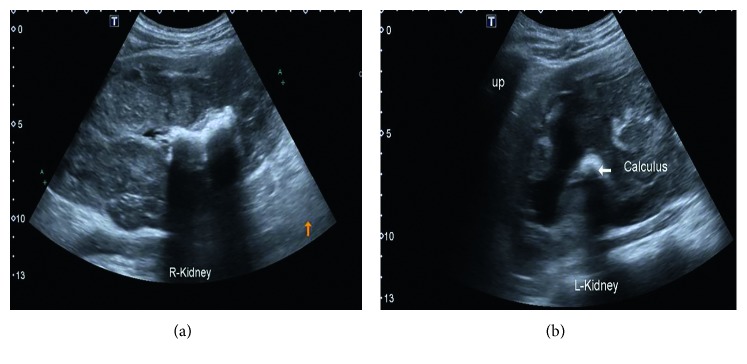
Ultrasound of the abdomen showed heterogeneous mass involving the right kidney and lower pole of the left kidney extending to the retroperitoneal space. The right kidney measured 17.1 cm, and the left kidney measured 14.6 cm. Both kidneys had loss of normal configuration. There was presence of staghorn calculi within the right kidney and calculus at the upper pole of the left kidney.
